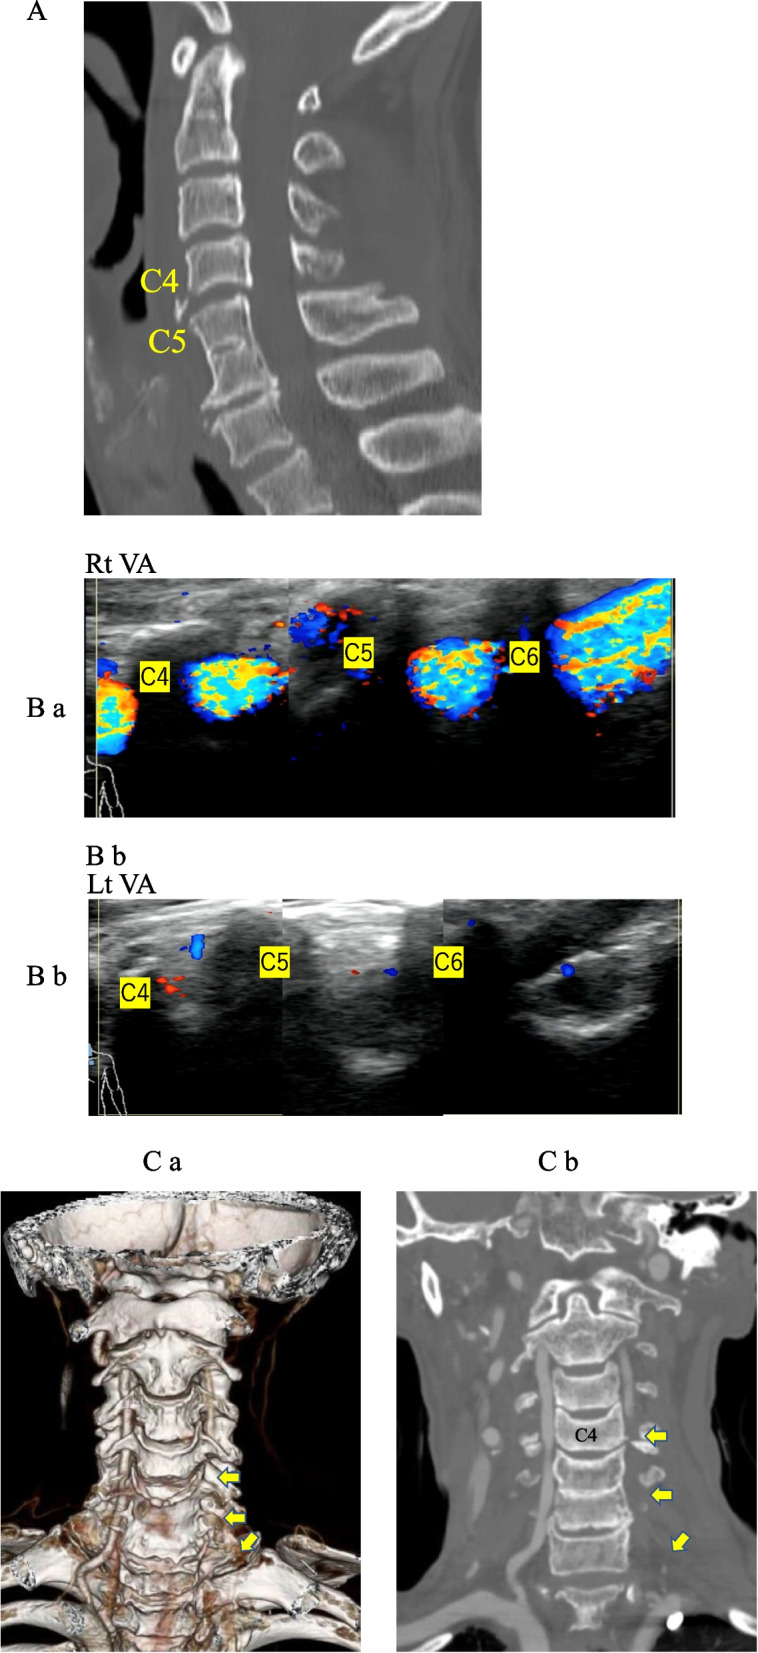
A CT shows a teardrop fracture at C4, suggesting hyper-extension injury. B ab. US shows no occlusion of the vertebral artery (VA) on the right side, but no flow of VA on the left-side. C ab. CTA shows no occlusion of the vertebral artery (VA) on the right side, but no flow of VA on the left side. CTA shows occlusion and flow of VA on the left side but not below C4 level (arrows), but this was not seen by US
